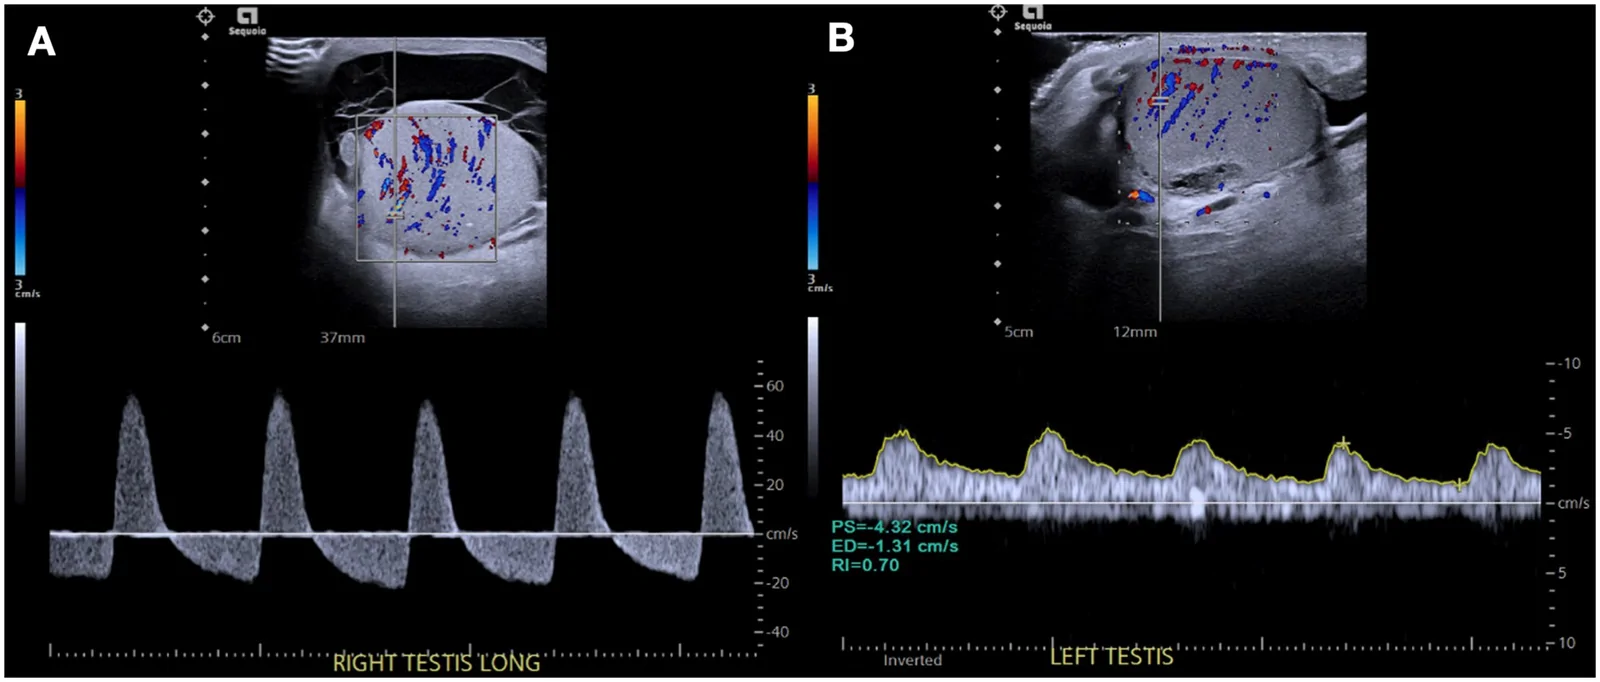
Initial spectral Doppler ultrasound. Images were obtained using a Siemens ACUSON Sequoia ultrasound machine with an 18L6 linear transducer and testicular preset. (A) Pulsed-wave spectral Doppler of the right testis, obtained with a gate size of 1.0, PRF 5208 Hz, and filter setting 40, demonstrating a high-resistance arterial waveform with reversal of diastolic flow and RI >1.0. (B) Pulsed-wave spectral Doppler of an intratesticular artery in the left testis, obtained with a gate size of 1.0, PRF 2000 Hz, and filter setting 40, showing preserved forward diastolic flow with PSV 4.32 cm/s, EDV 1.31 cm/s, and RI 0.70.
